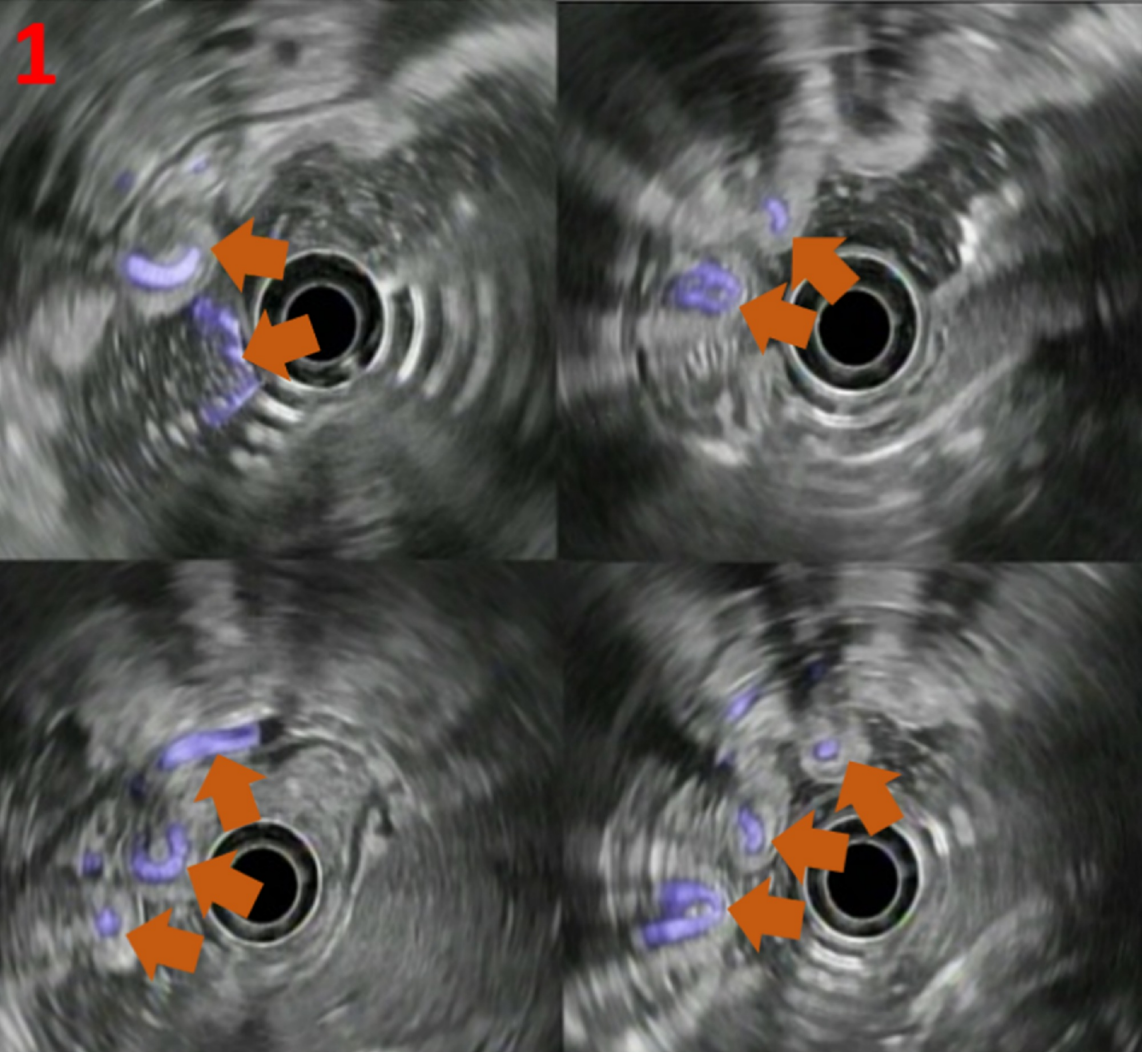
Esophageal ultrasound of varices Esophageal ultrasound with color Doppler signal shows varices (orange arrows) as demonstrated by serpiginous veins (purple fill)
